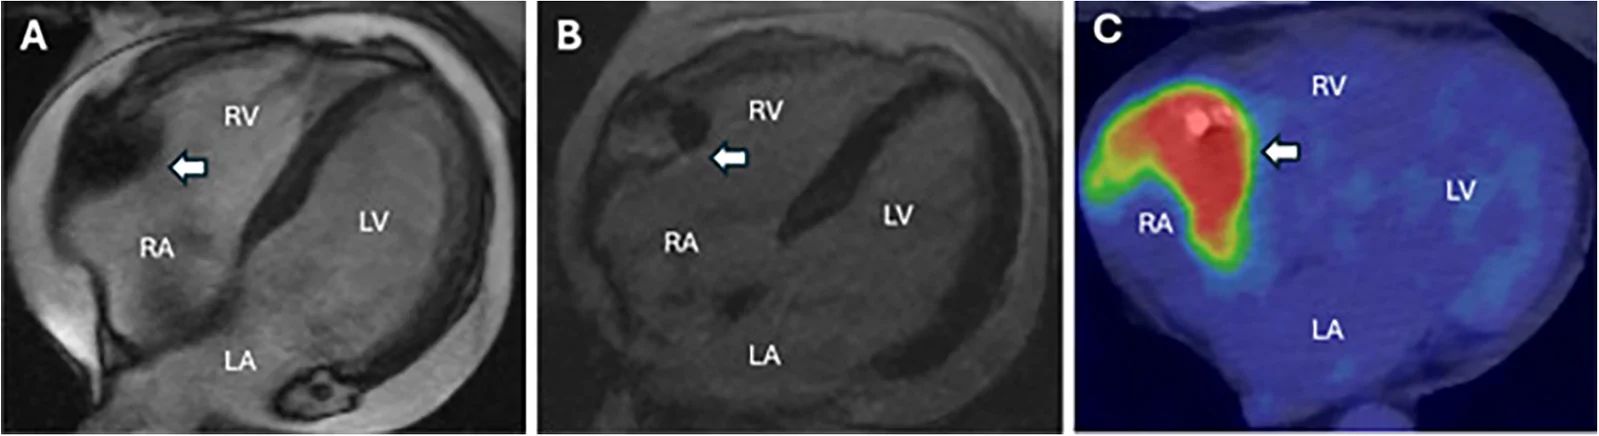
(A,B) Cardiac MRI reveals an irregular 5  ×  5  ×  2 cm mass centered in the right atrioventricular groove (arrow), extending into the posterior and medial right atrial wall and along the right ventricular free wall. The lesion is T2 hyperintense with mixed late gadolinium enhancement and associated moderate pericardial effusion with fibrinous strands. (C) Fluorodeoxyglucose positron emission tomography–computed tomography demonstrates intense radiotracer uptake within the right atrium (arrow) corresponding to the MRI-defined mass, confirming metabolically active myocardial infiltration by extranodal NK/T-cell lymphoma.
